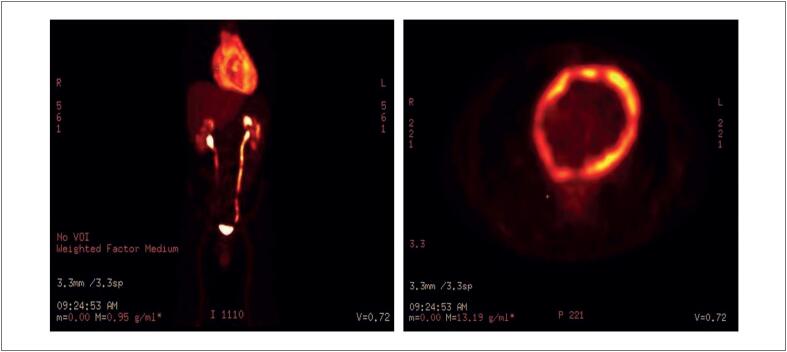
Tomografia por emissão de pósitrons/Tomografia Computadorizada usando fluorodesoxiglicose (^18^F-FDG-PET/CT) revela atividade hipermetabólica do pericárdio, com um valor de captação padronizado máximo (SUVmax) de 12,8 g/mL.
